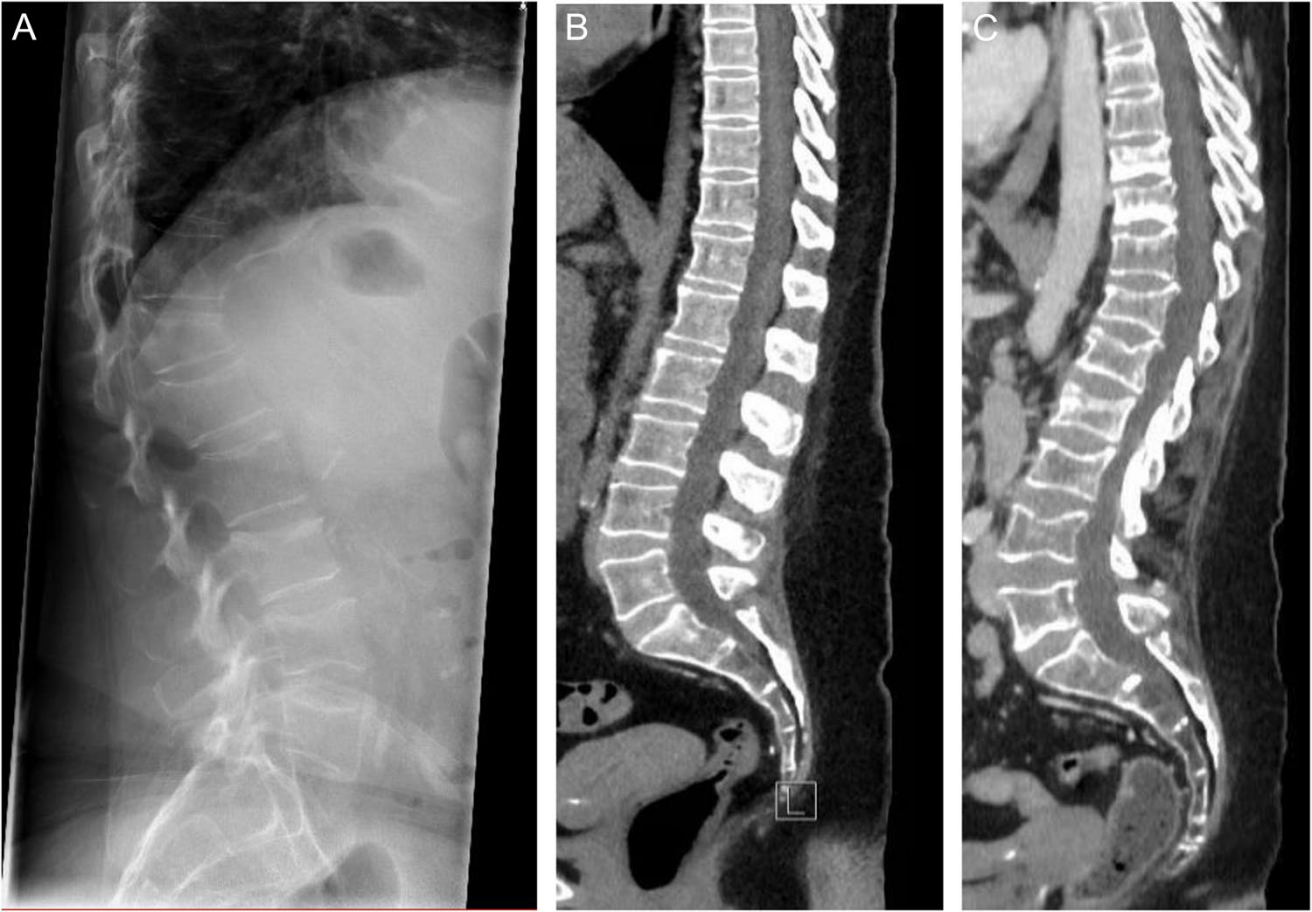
Imaging of vertebral fractures. A Plain X-ray of the lumbar spine shortly after fourth vertebral fracture. B Sagittal reconstruction of spinal computed tomography images performed 2 years prior to the first fracture. C Sagittal reconstruction of spinal computed tomography images 1 year after the first fracture
